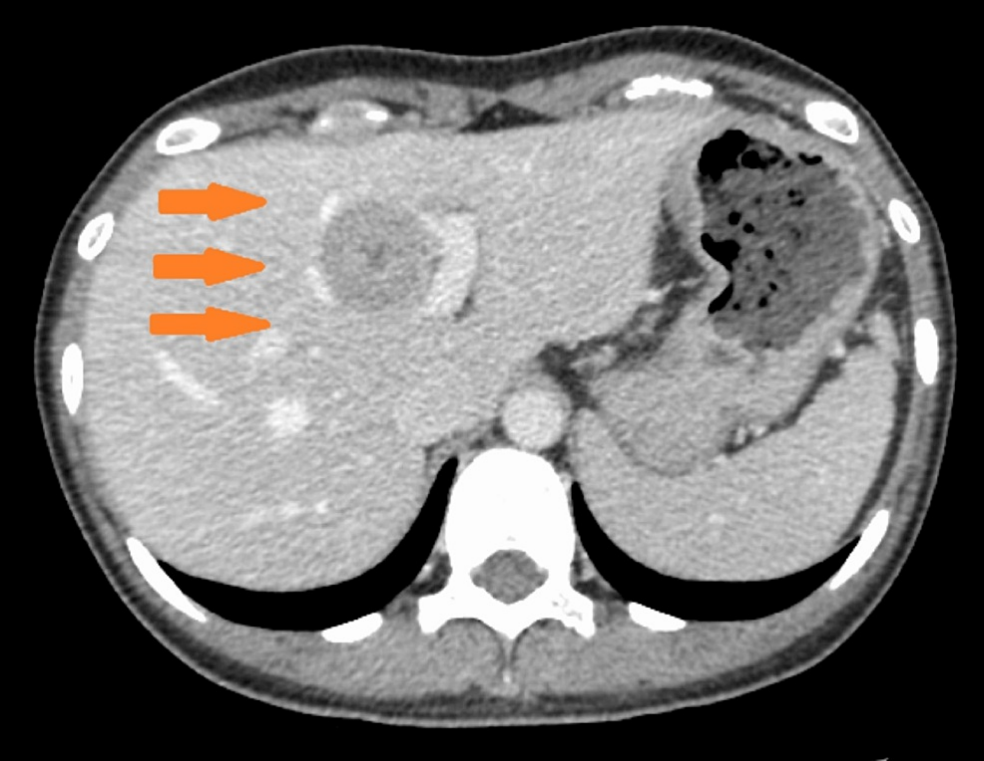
CT scan of the abdomen. Liver tumor at initial diagnosis.
